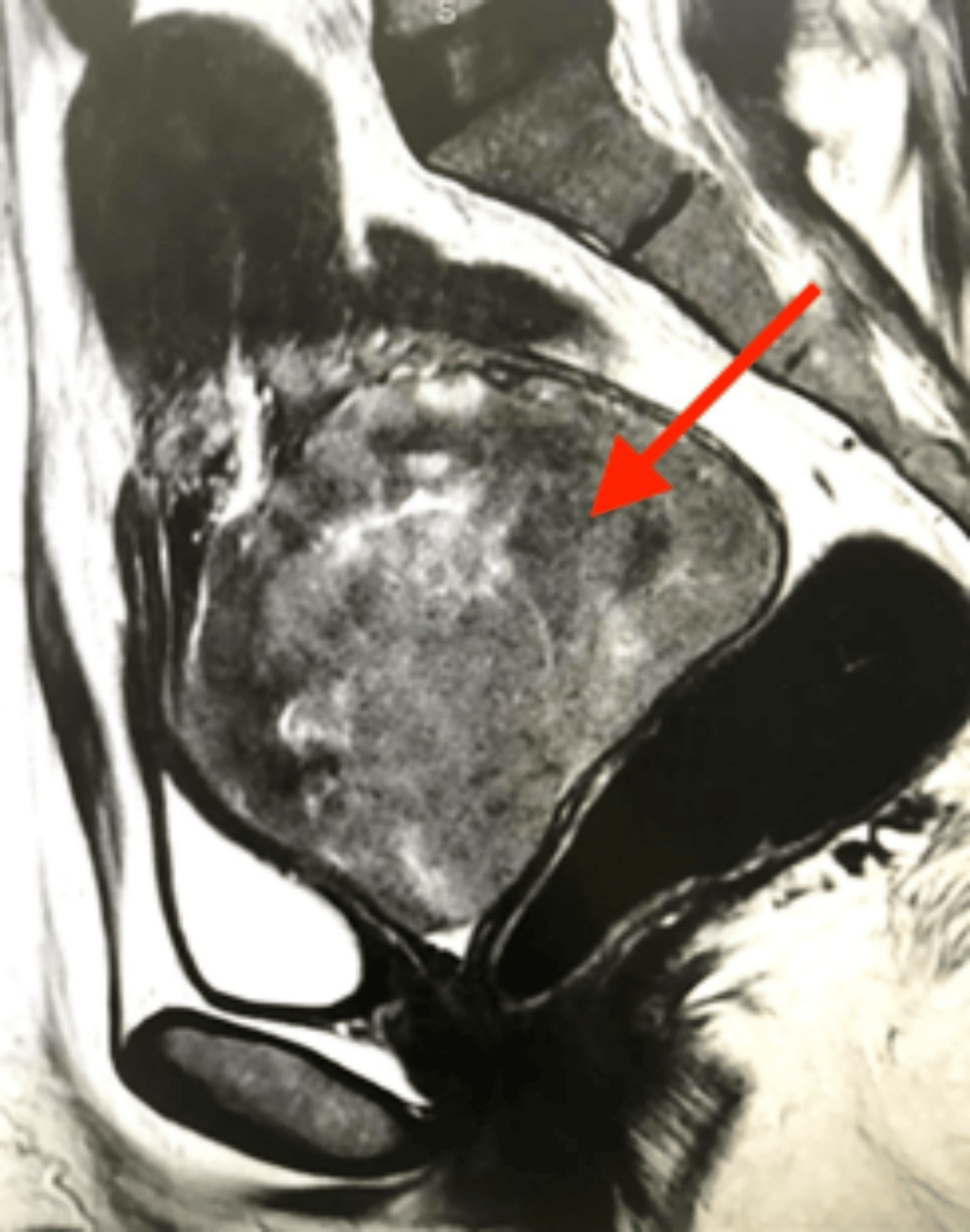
Sagittal section of T2-weighted MRI of the pelvis showing an exophytic tumour mass arising from the posterior cervix (red arrow) and filling the upper vagina measuring 9.3 cm.
